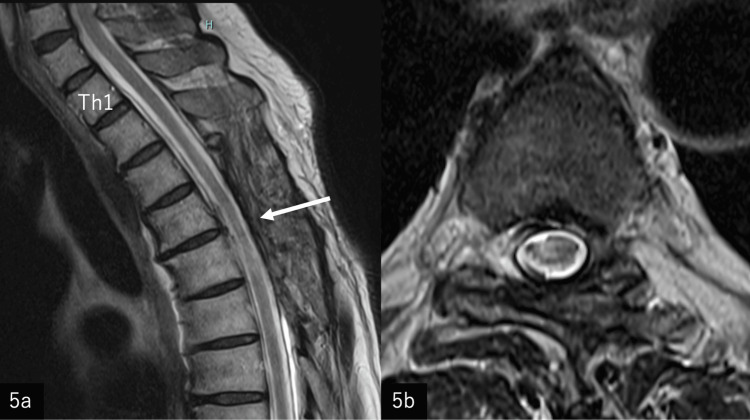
Post-operative MRI 5a: Postoperative T2-weighted midsagittal MRI two years after surgery. The anterior deviation of the spinal cord has improved, and the spinal cord is now centrally located within the dural canal (arrow). 5b: Postoperative T2-weighted axial MRI at the T4-T5 level. The anterior deviation of the spinal cord has improved, with the spinal cord now centrally located within the dural canal. Signal intensity changes are observed in some areas of the spinal cord.
